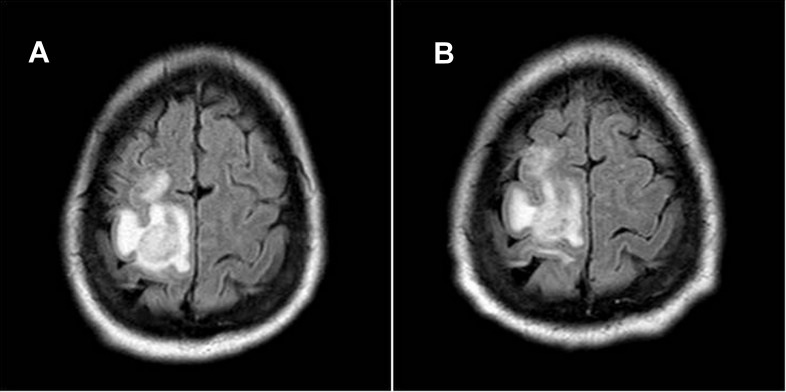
Brain MRI of patient 2 at presentation showed an irregular ring-enhancing lesion in the right frontoparietal region with maximal transverse dimension of 2.2 cm (
